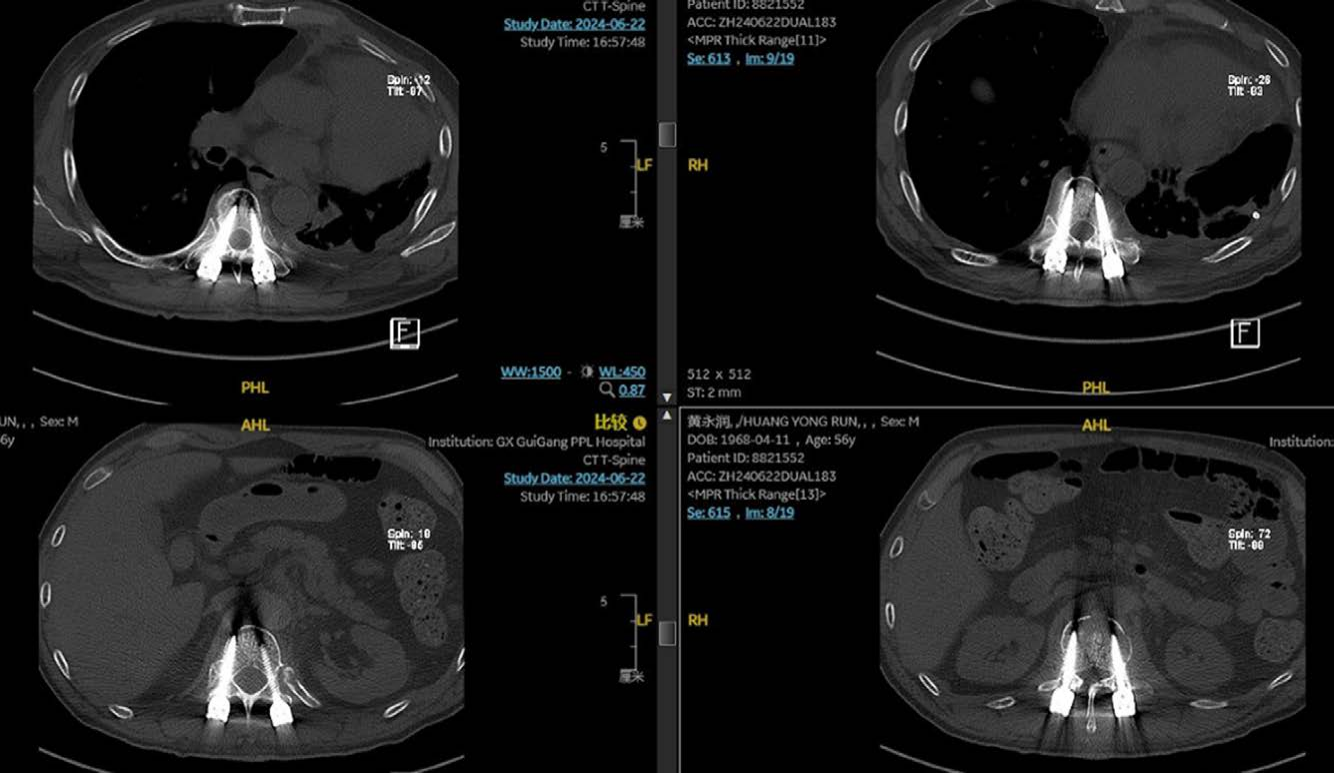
Thoracolumbar computed tomography (CT) on postoperative day 9. CT = computed tomography.
